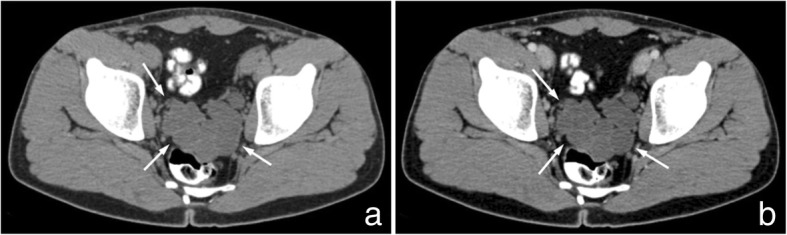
Axial CT image of the pelvis (a) depicts a large lobulated multicystic lesion of left seminal vesicle; the cystic lesion does not show enhancement on contrast-enhanced axial CT image (b) (arrows)
